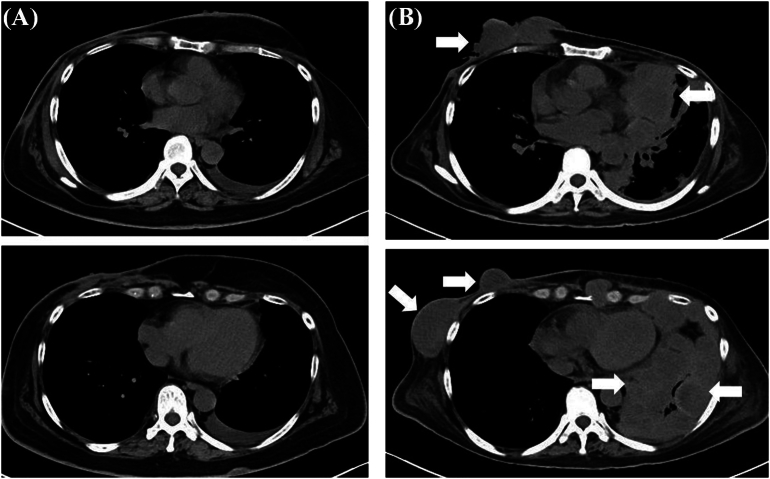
Simple Thoracic CT; (A) 4 weeks and (B) 17 weeks after operation of right local recurrence. The right chest wall mass and left pleural tumor grew rapidly, and after 17 weeks, the left thoracic cavity was almost 70 % occupied by a pleural mass and the right chest wall mass grew to 10 cm in size.
